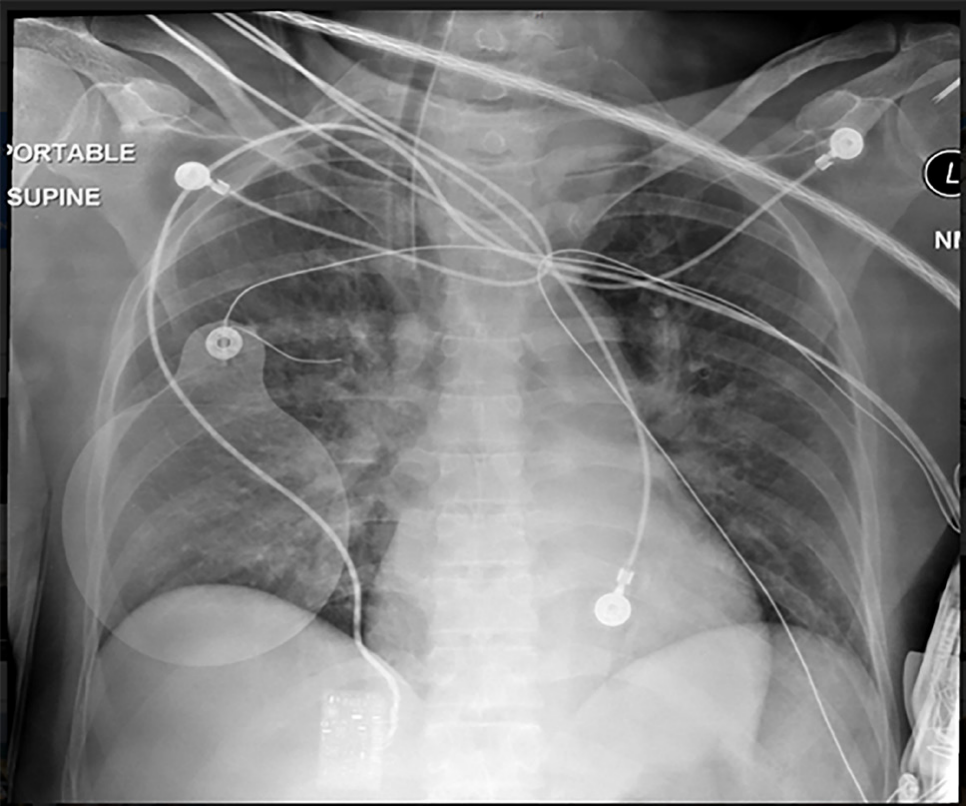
Anterior-posterior bedside chest radiograph.
